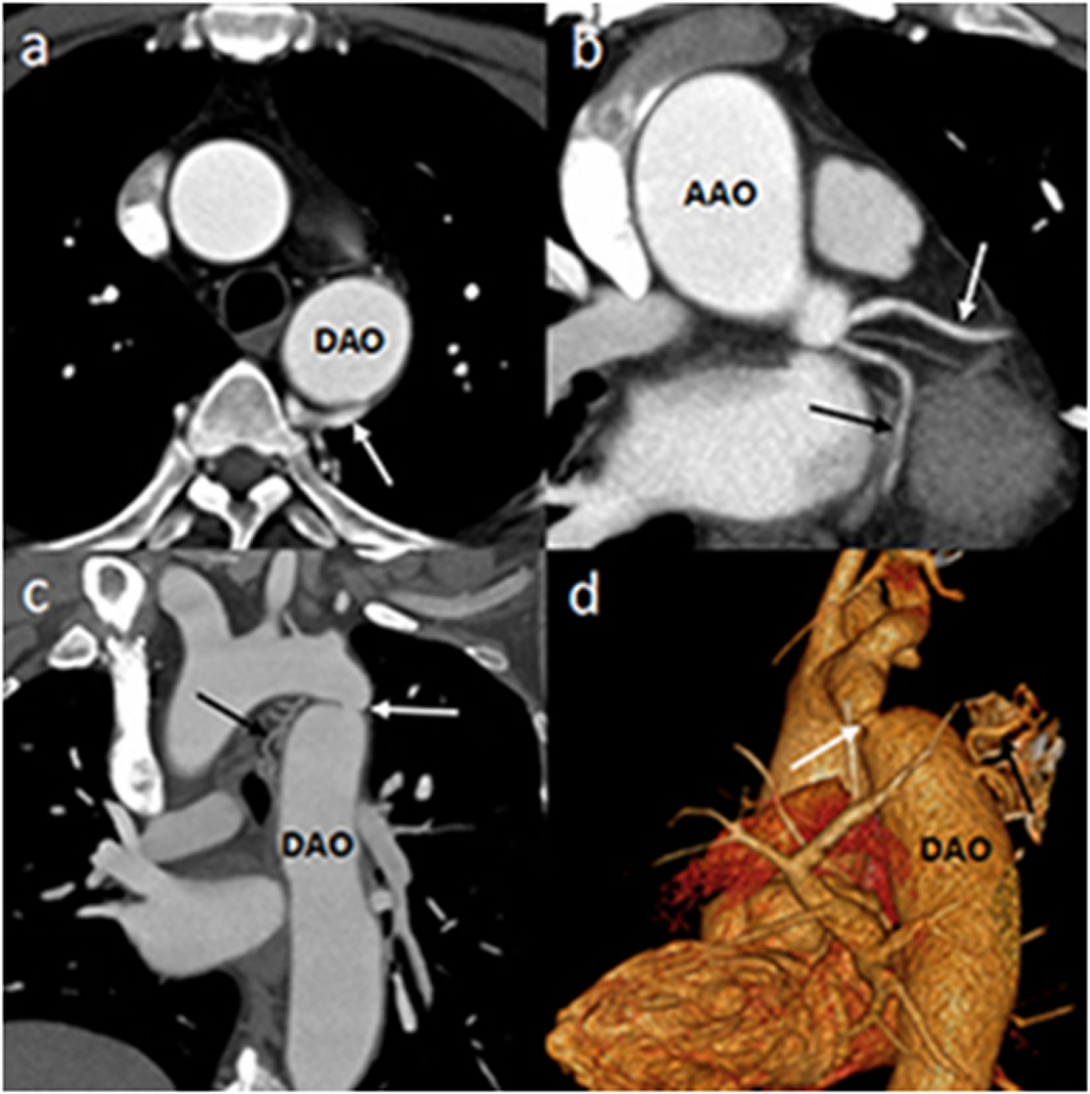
DSCT images of a 43-year-old man with coarctation of the aorta. Axial image (a) shows the dilated collateral vessels from DAO (white arrow). Axial multiplanar reformatted image (b) shows LAD (white arrow) and LCX (black arrow) origin from left coronary sinus respectively. The multiplanar reformatted image (c) and volume rendering image (d) show the site of coarctation (white arrow) and the collateral vessels (black arrow). *Abbreviations*: DSCT, dual-source computed tomography; DAO, descending aorta; AAO, ascending aorta.
